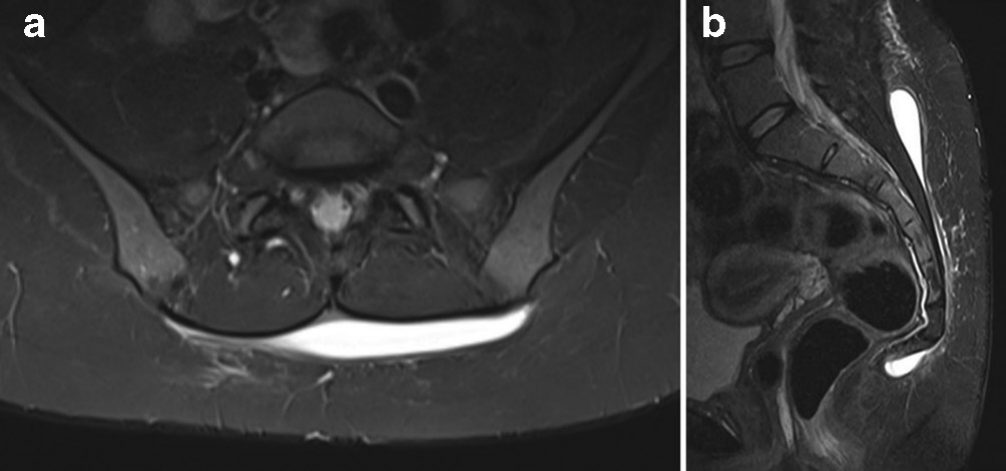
(a) Axial and (b) sagittal initial short tau inversion recovery (MRI) of retrosacral Morel-Lavallée lesion following three percutaneous aspirations. There is a high signal collection seen at the deep margin of the subcutaneous fat, but superficial to the fascia overlying the paraspinal musculature. On the sagittal sequence distal extension of the lesion to the level of the coccyx is identified.
